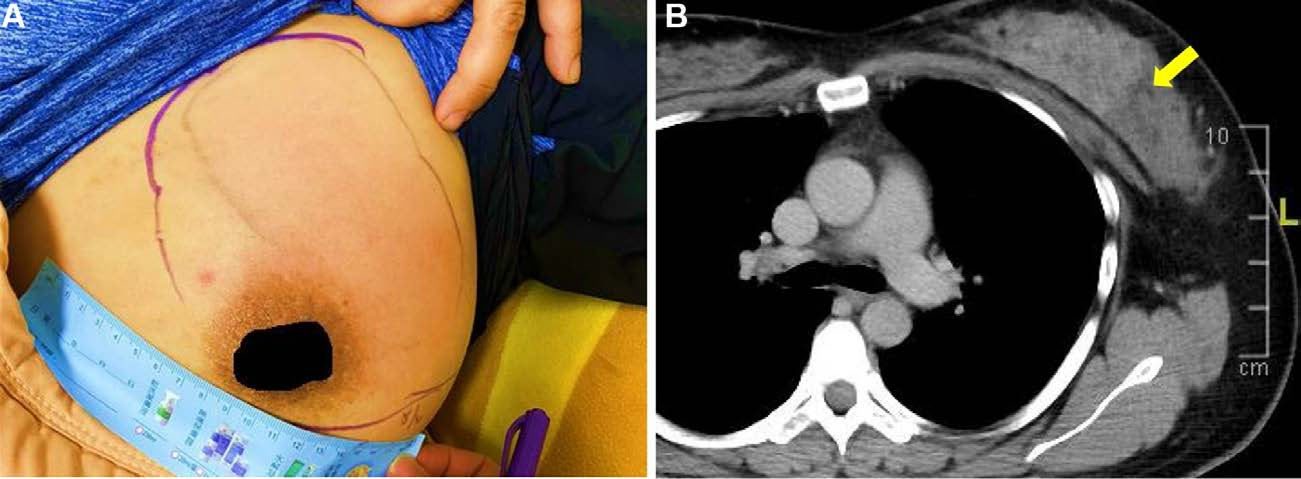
(A) A 33-year-old female patient presented with painful erythematous swelling on the left breast. (B) CT scan demonstrated heterogenous inflammation in the breast gland tissue (indicated by arrow).
